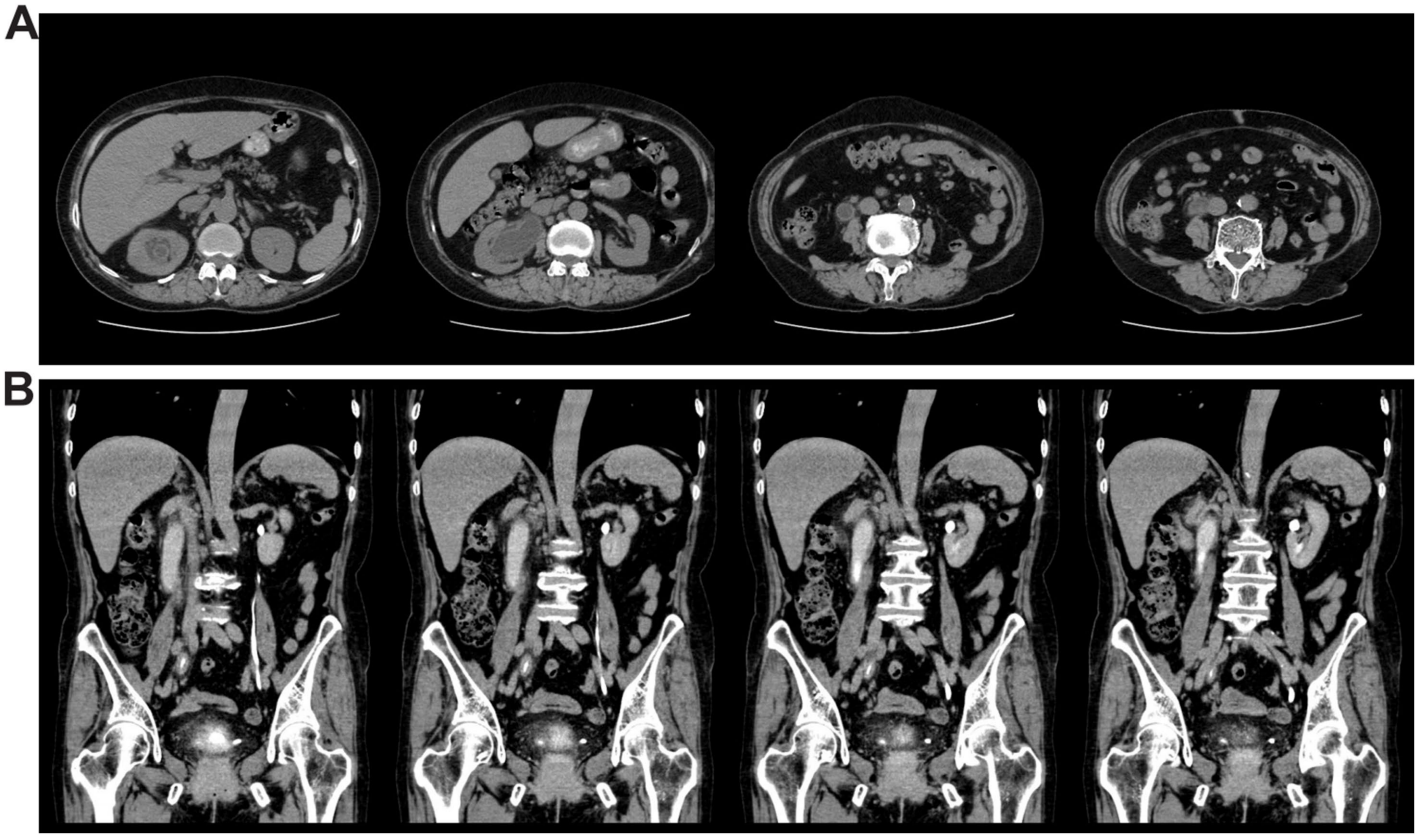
CT images showed thickening and hydronephrosis of the right kidney and right ureter, as well as inflammation of the bladder. (A) Whole abdominal CT of the patient. (B) CT urography of the patient.
